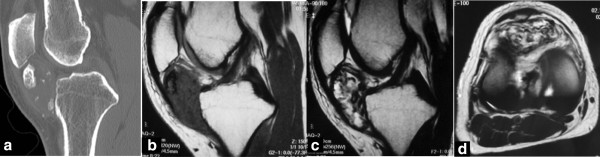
CT and MRI revealed the multiple tumors as ossification in the infrapatellar fat pad. a CT MPR image b Sagittal T1-weighted MRI c Sagittal T2-weighted MRI d Axial T2-weighted MRI.
